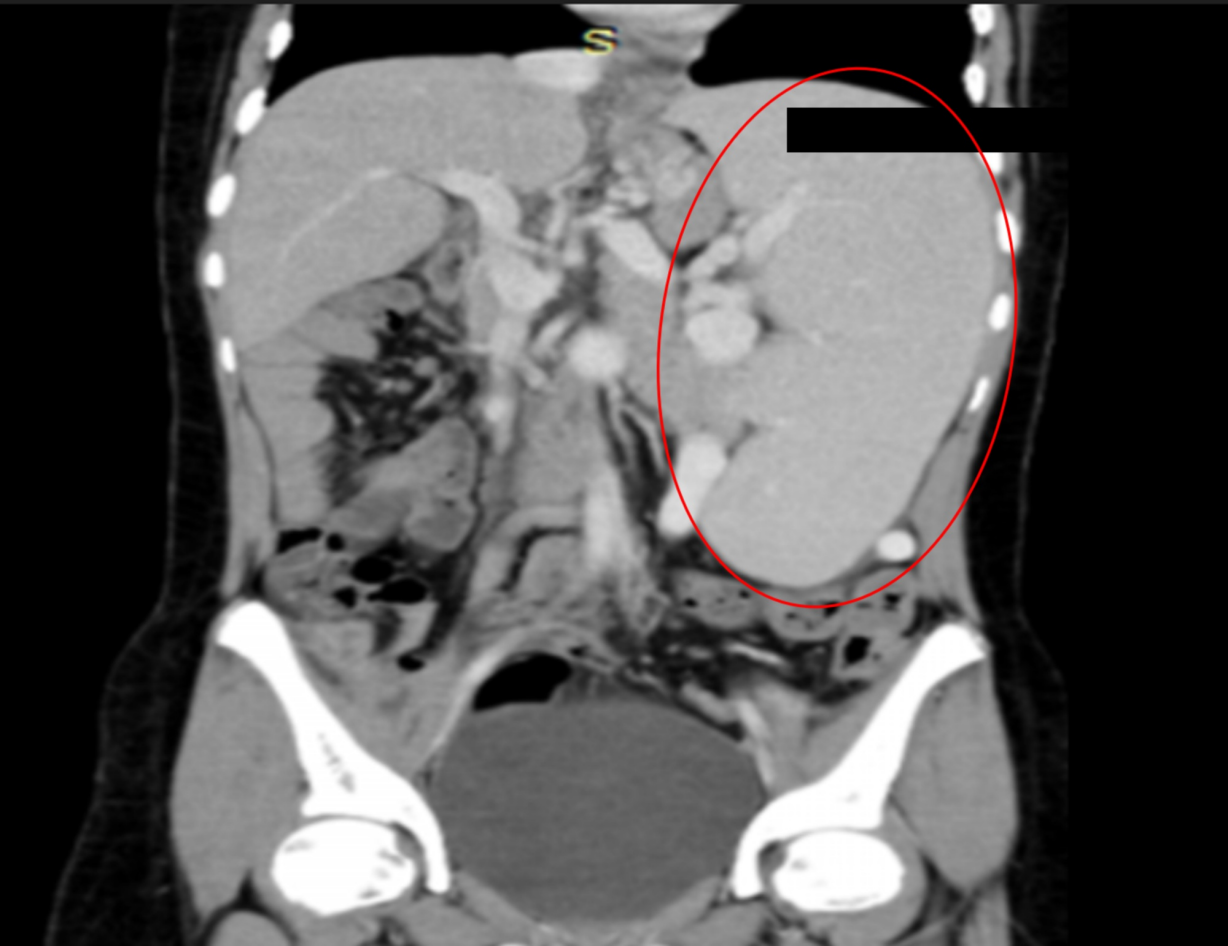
CT scan showing a dilated portal vein with splenomegaly and splenic varices.
